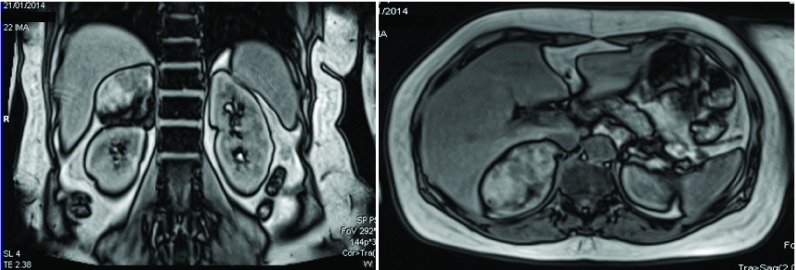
Triphasic abdominal MRI showing a right 7.0 × 6.0 cm adrenal incidentaloma. Left panel, longitudinal cut; right panel, transverse cut. Performed using SIEMENS Magneton Essenza 1.5 TESLA.
